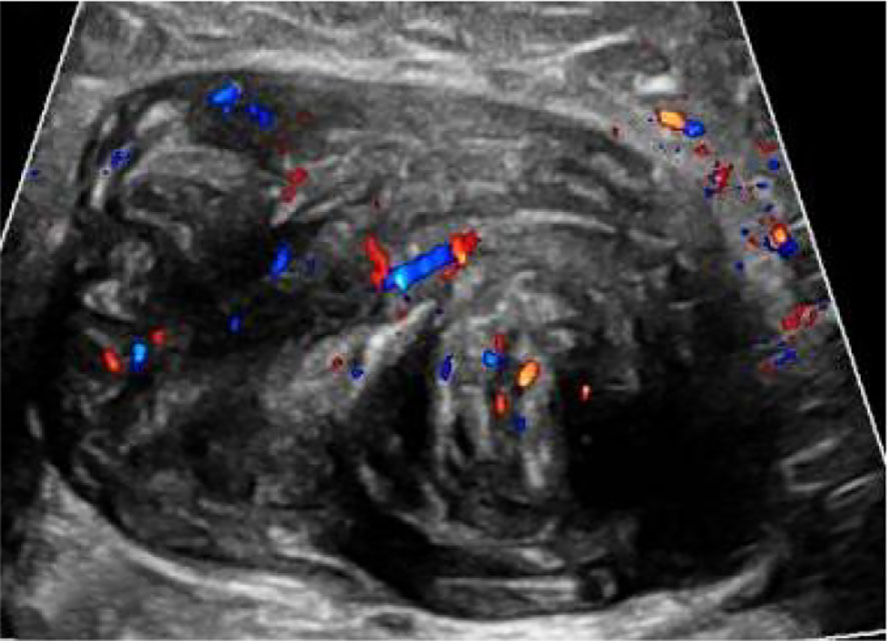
Breast ultrasound showed an irregular hypoechoic solid mass.
